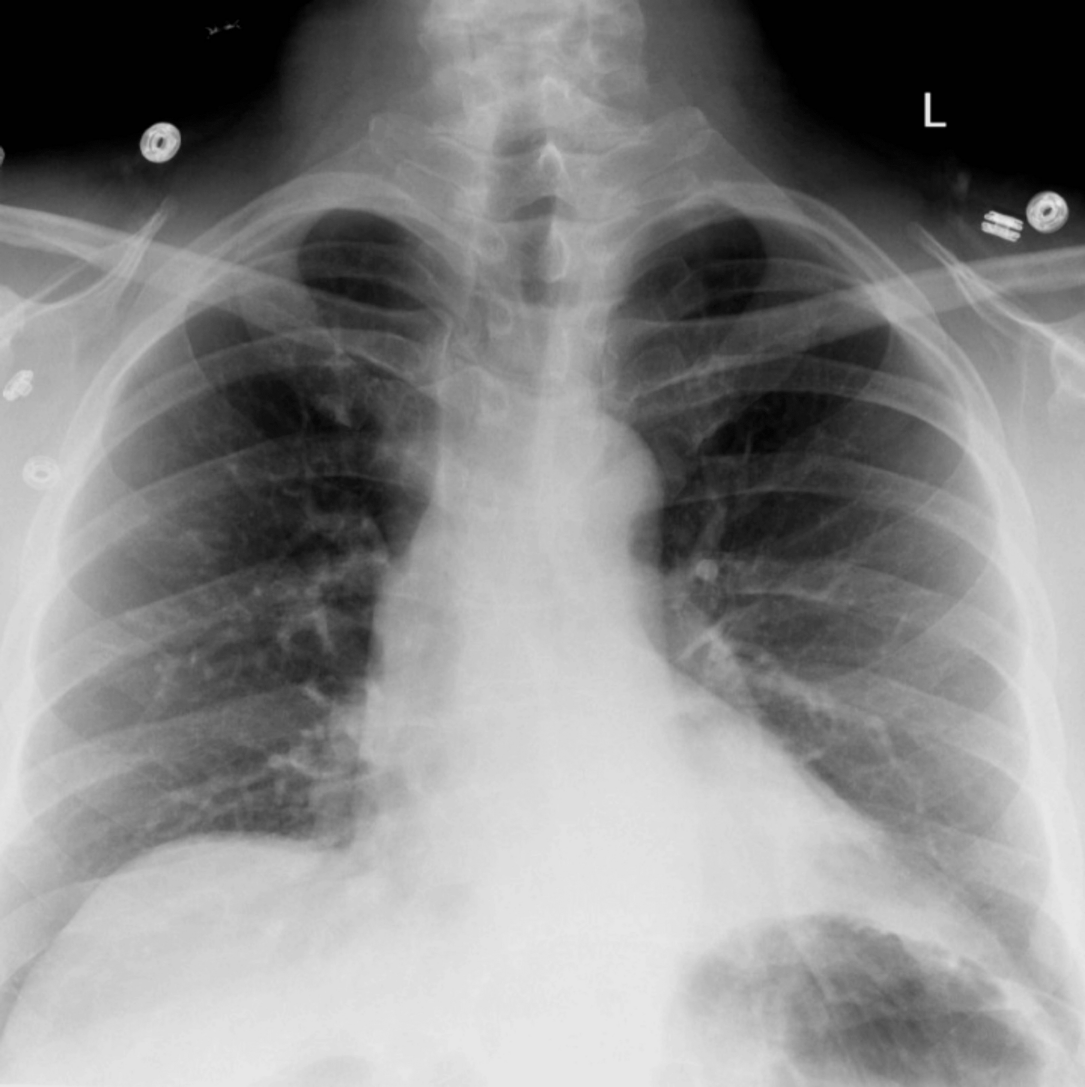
Chest X-ray obtained on postoperative day 1 after removal of the chest tube There is no evidence of a large pleural effusion or pneumothorax.
